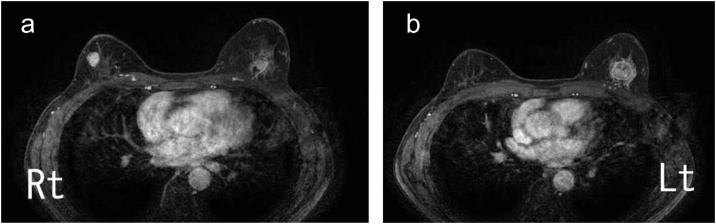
Contrast-enhanced breast magnetic resonance images at the first visit. a) In the right breast, an 18-mm, well-defined, marginal spicula-like lobulated tumor that is strongly enhanced from an early stage is observed. b) In the left breast, a 21-mm, well-defined circular tumor with irregular margins that is strongly enhanced from an early stage is observed.
